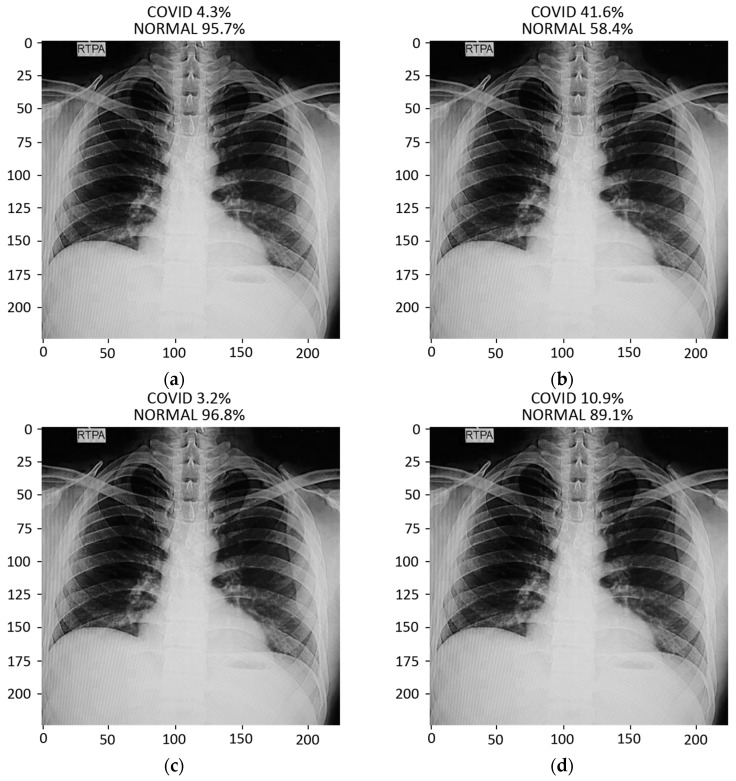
Results of prediction on a verified local Pakistani normal chest X-ray: (a) DenseNet-121; (b) VGG16; (c) MobileNet; (d) ResNet-50.
